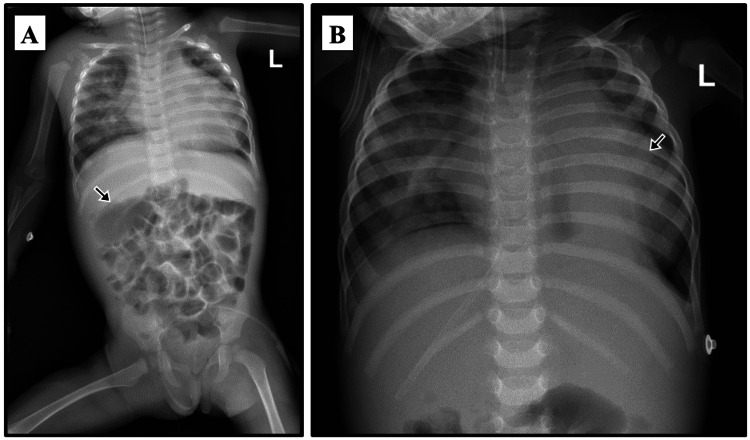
A plain X-ray of the chest and abdomen of the patient with cyclin-dependent kinase 13-related disorder. (A) The nasogastric tube (arrow) passes to the right side towards the right-sided stomach indicating the situs inversus partialis. (B) Right-sided lung infiltrate indicating pneumonia with an enlarged cardiac shadow indicating cardiomegaly (arrow).
